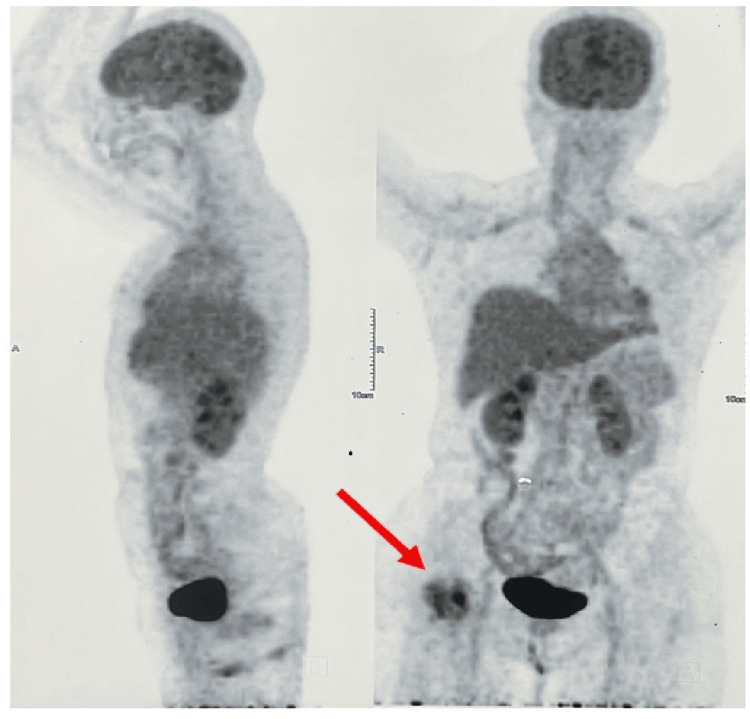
18-fluorodeoxyglucose (FDG) PET CT scan The red arrow mark shows tracer uptake with mixed density in the proximal femur area indicative of a neoplastic etiology.
